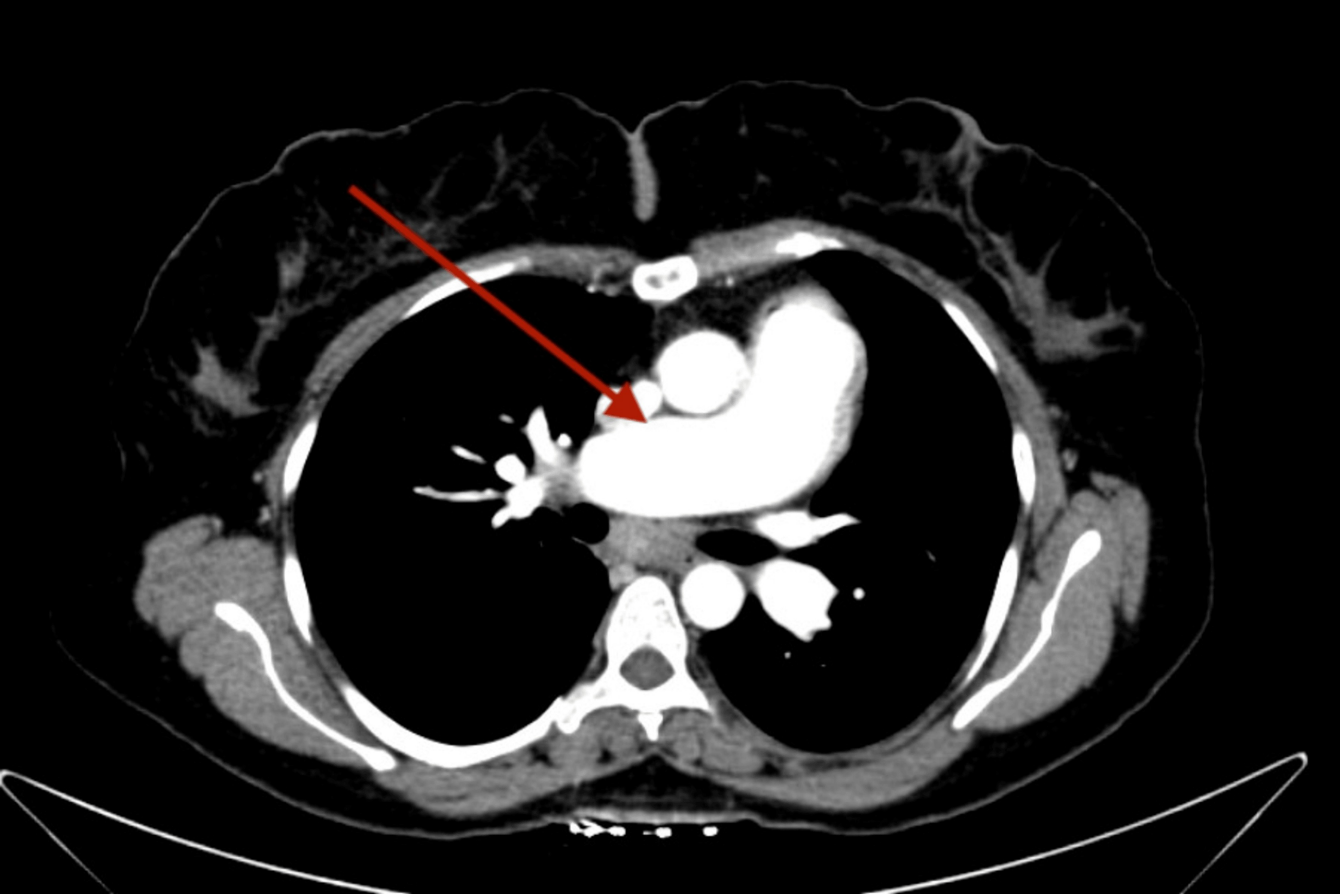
CT chest showed dilated pulmonary trunk
